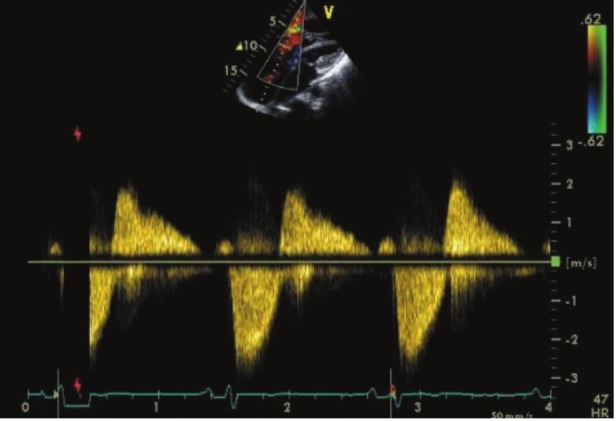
Continuous-wave Doppler demonstrating a daggershaped pattern in a patient with severe tricuspid regurgitation due to carcinoid heart disease, with early peak pressure and a rapid decline. In addition, the patient had marked failure of coaptation (FOC) resulting from severely thickened and retracted tricuspid valve leaflets due to underlying carcinoid disease (image courtesy of Fox and Khattar).^5^
